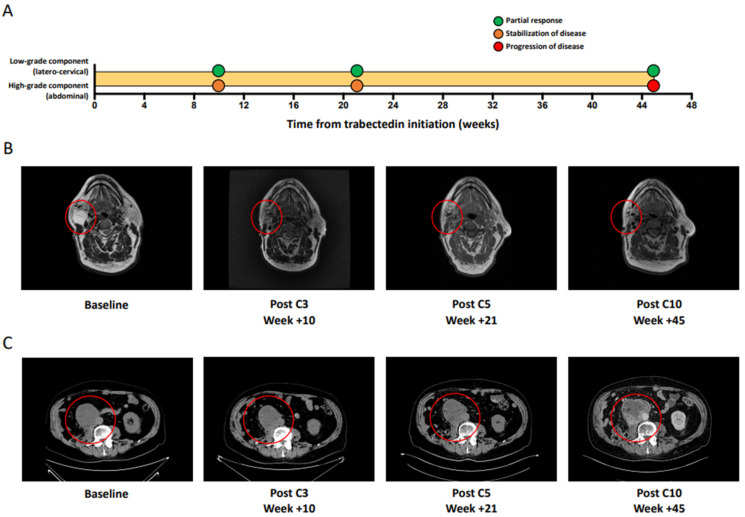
Anecdotal mixed response to trabectedin in a patient with double dedifferentiated liposarcoma (DDLPS) component. (A) shows the different dynamic of response to trabectedin of the low-grade, laterocervical component (low-grade DDLPS) vs. the high-grade, abdominal component (high-grade DDLPS). (B) (T2 weighted magnetic resonance imaging) and (C) (computerized tomography scan) show the RECIST tumor response at specific time-points of the low-grade, laterocervical component and of the high-grade, abdominal component, respectively.
